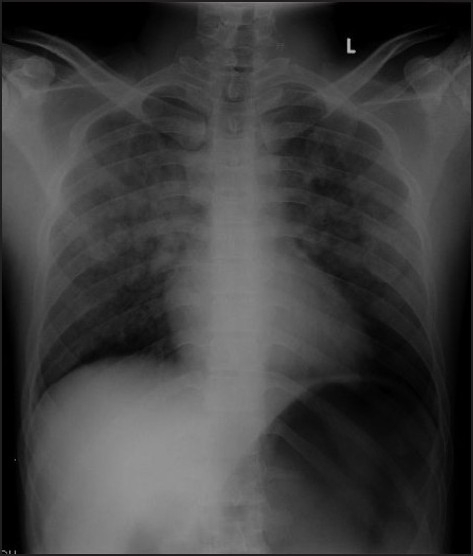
Thirty-minute post-operative chest X-ray, showing diffuse infiltrates more in mid-zone perihilar region, obvious air shadow in gastric fundus
